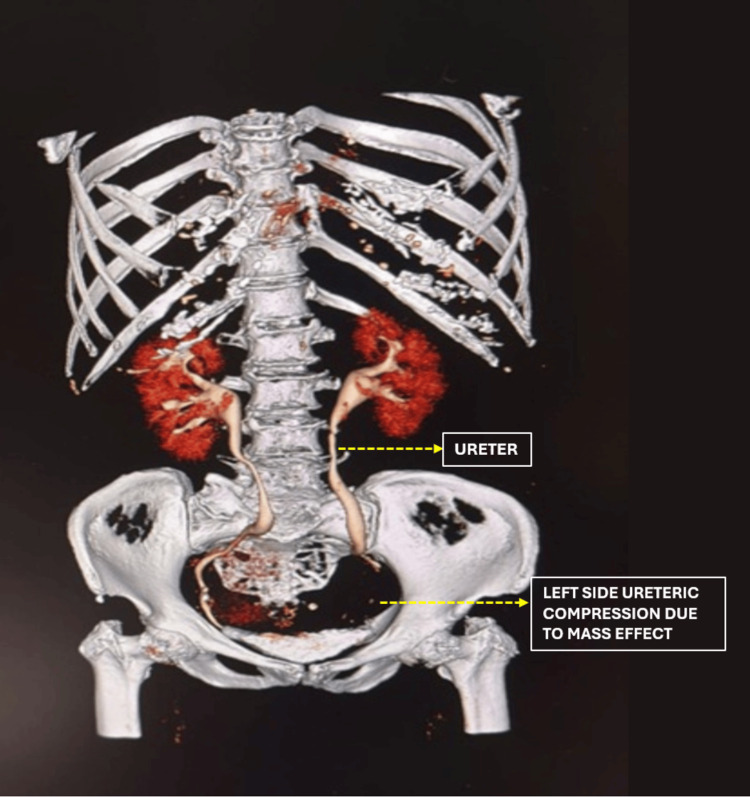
Case 2: Three-dimensional CT volume rendering of contrast-enhanced CT of the abdomen and pelvis Bilateral ureteric displacement is clearly demonstrated as both ureters course alongside the enlarged fibroid uterus before entering the pelvis. The dominant lower uterine segment and cervical fibroid (11 × 5.7 × 7.2 cm) causes medial displacement and dilatation of both ureters. This three-dimensional reconstruction formed the basis for bilateral prophylactic DJ stenting. DJ: Double-J.
